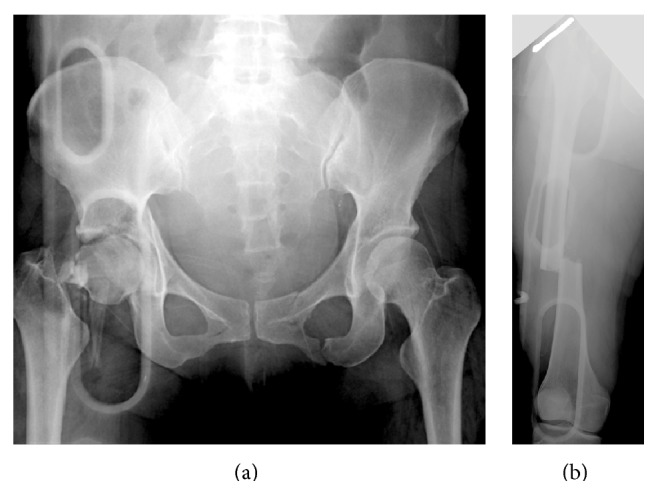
Initial radiographs of the fracture sites. (a) An anteroposterior pelvic radiograph showing an AO type B3 fracture of the right acetabulum and AO type C3 fracture of the right femoral neck. (b) An anteroposterior radiograph showing an AO type A3 fracture of the right femoral shaft with a Gustilo type 2 open wound.
